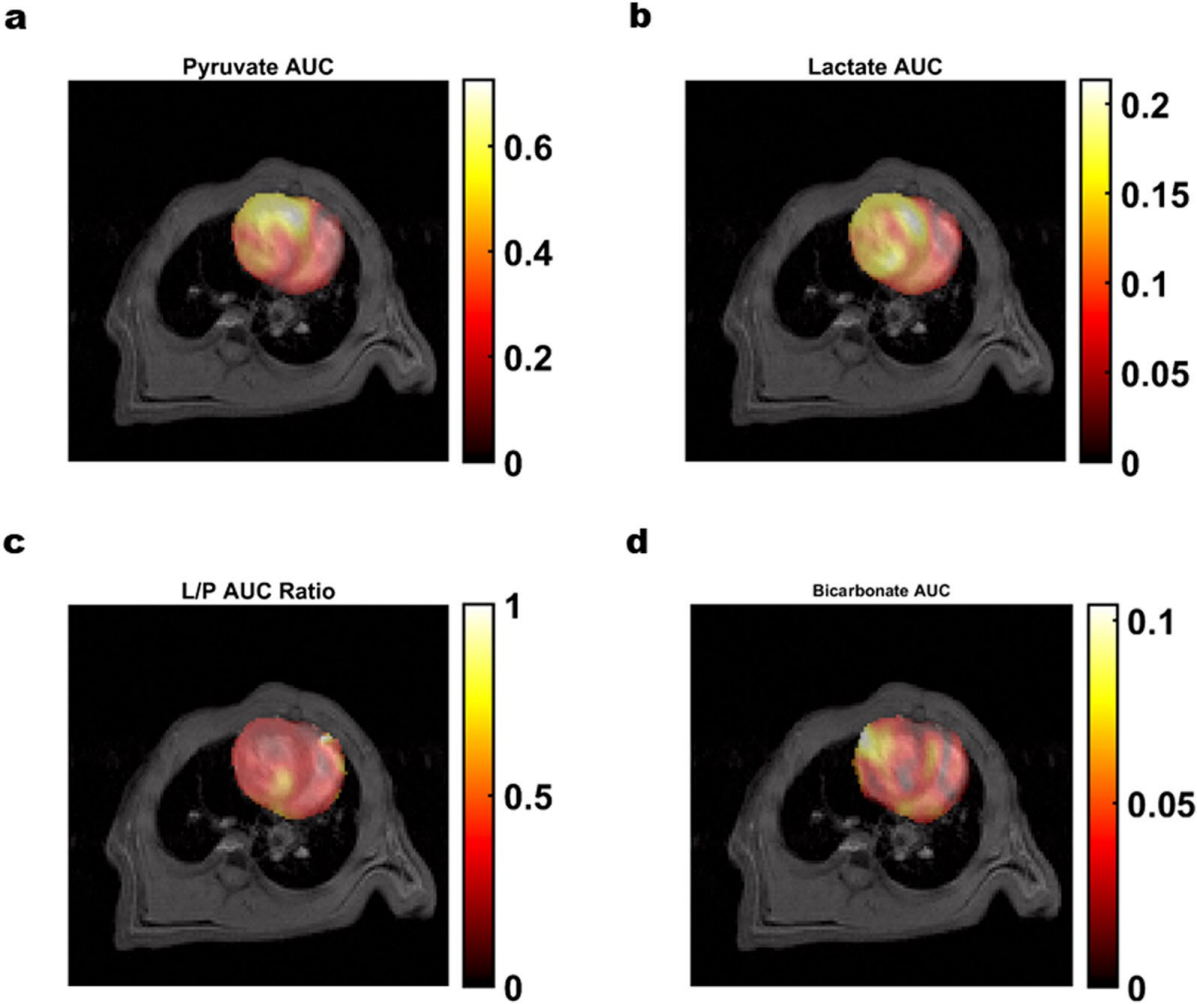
^13^C images of pyruvate (a), lactate (b), lactate/pyruvate (L/P) area under the curve (AUC) ratio (c), and bicarbonate (d) in the LV measured using dynamic HP [1-13C]pyruvate MRI. Metabolite images (color) are overlaid on T1-weighted axial images (grayscale) for anatomical reference. Reproduced with permission from Elsevier Ltd (License Number: 5324651196851). Magn Reson Imaging. 2020 May;68:9–17
